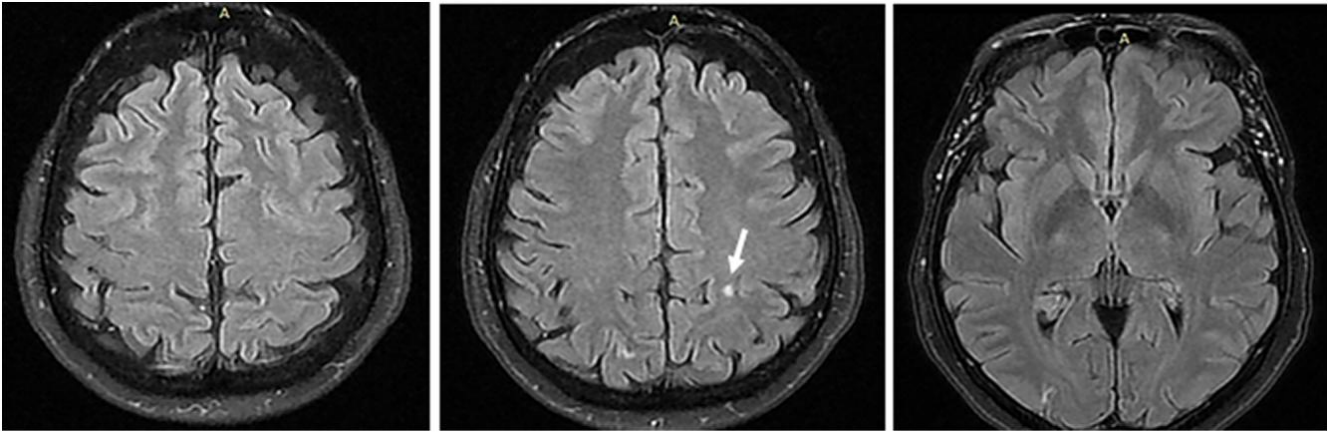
Brain MRI 3 months after the episode, with complete resolution of the previous reported abnormalities, except for a small gliotic lesion (4 mm) in the white matter of the left posterior superior parietal lobe (arrow).
